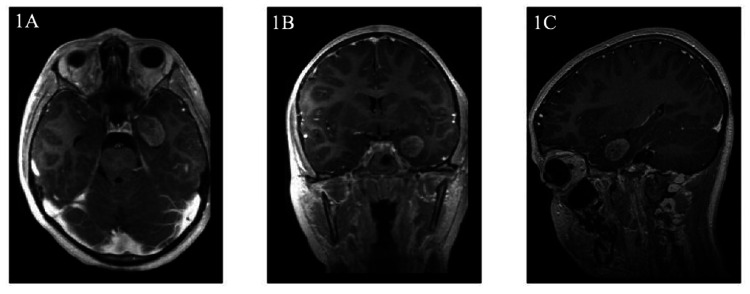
T1-weighted MRI of patient's intracranial tumor. T1-weighted MRI characterizing the mass as a 1.7 cm x 1.7 cm x 2.6 cm left medial temporal lobe enhancing lesion in the (A) transverse, (B) coronal, and (C) sagittal views.
